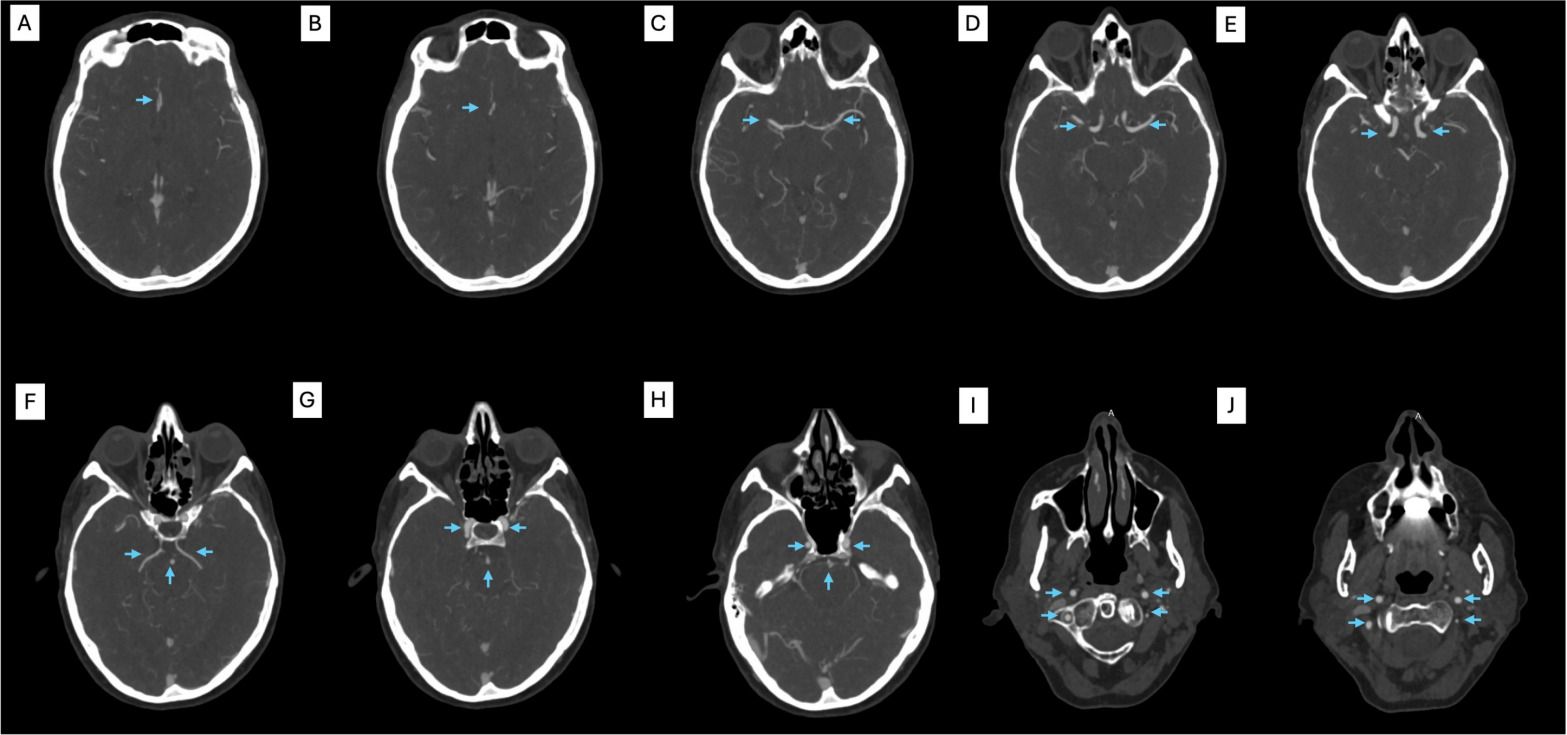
CT angiography showing no flow-limiting stenosis in the anterior or posterior circulation (A, B) Anterior cerebral arteries. (C, E) Middle cerebral arteries and internal carotid arteries. (F) Posterior cerebral arteries and basilar artery. (G, H) Carotid arteries and basilar arteries. (I, J) Carotid arteries and vertebral arteries.
